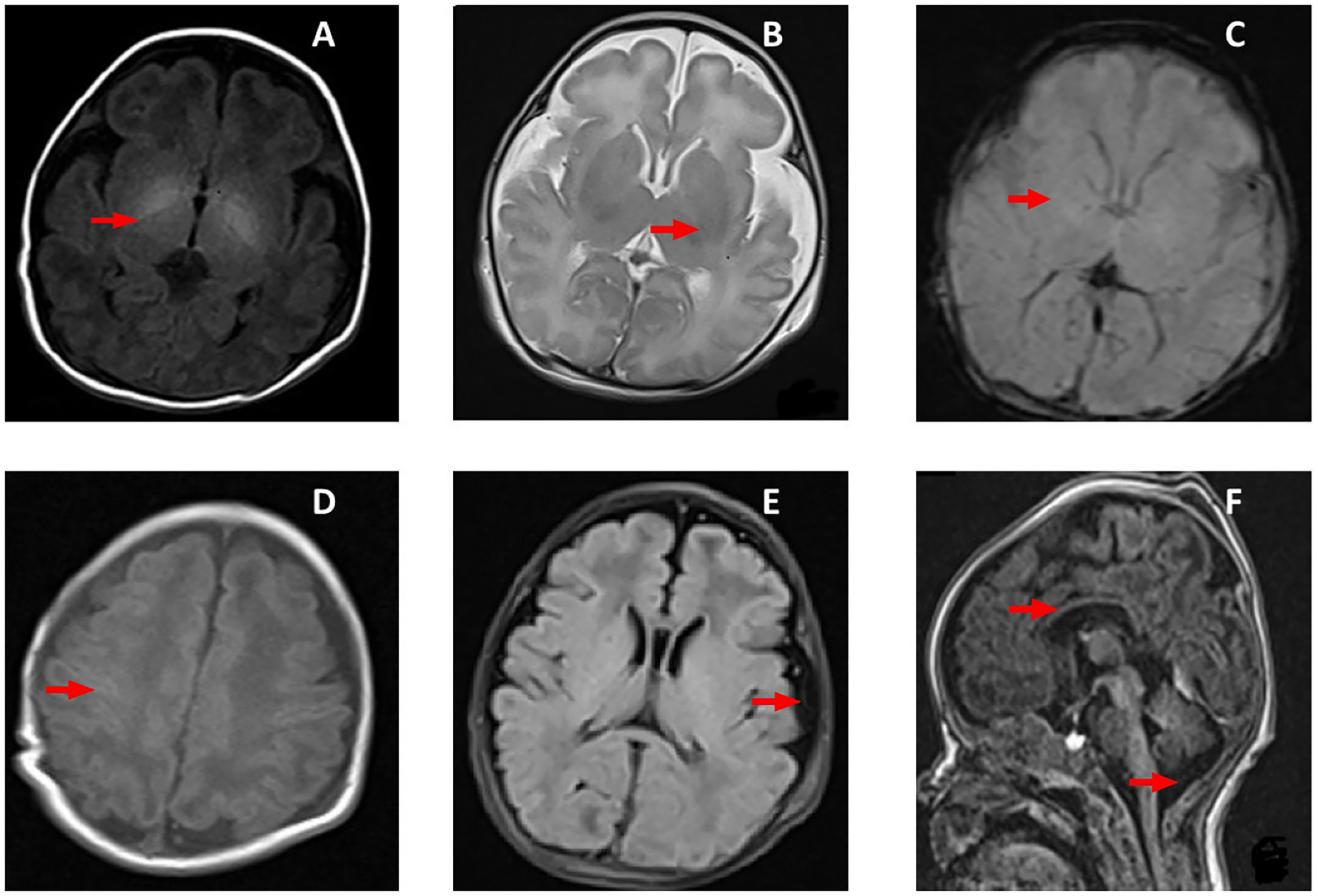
MRI images of the patient done on Day 5 of life. (A) Axial T1 image showing no myelination in posterior limb of internal capsule and high signals in basal ganglia. (B) Axial T2 image showing no myelination in posterior limb of internal capsule and normal signals in basal ganglia. (C) Axial gradient image showing no blooming and normal signals in basal ganglia. (B, C) Suggests absence of hemorrhage and vasogenic edema. (D) Image shows no myelination in perirolandic gyri. (E) Axial FLAIR image showing simplified cortical patterns and prominent sulcal spaces, and (F) Sagittal T1 images show thin corpus callosum and prominent cisterna magna.
